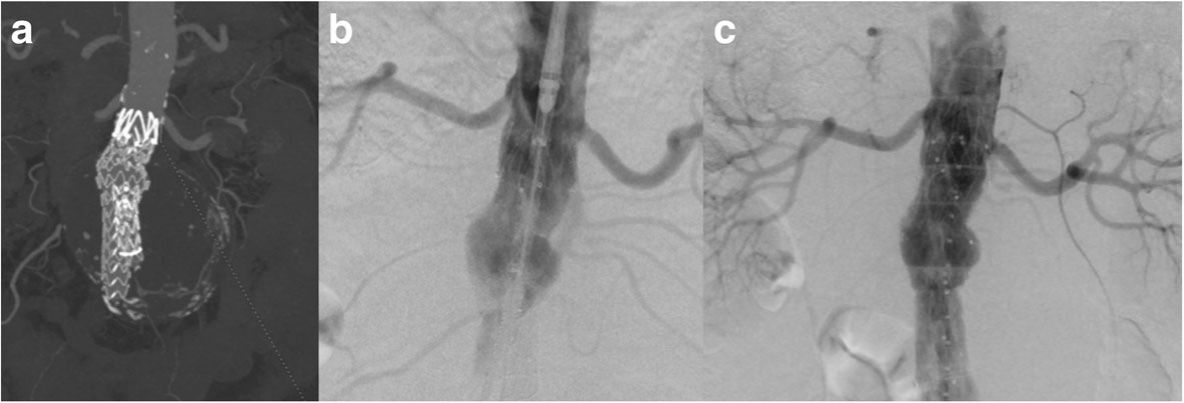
a Coronary reconstruction of CTA scan confirming deployment of the graft in a caudal position led to sac expansion in the follow-up period. b Angiogram confirmed the low graft position. c A cuff was deployed in an immediate infrarenal position to prevent any further sac expansion
